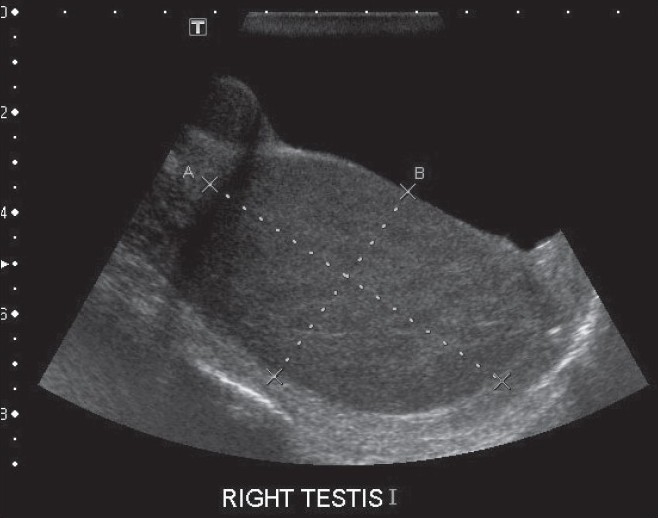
Ultrasound of the right testis showing homogenously enlarged testicular parenchyma
